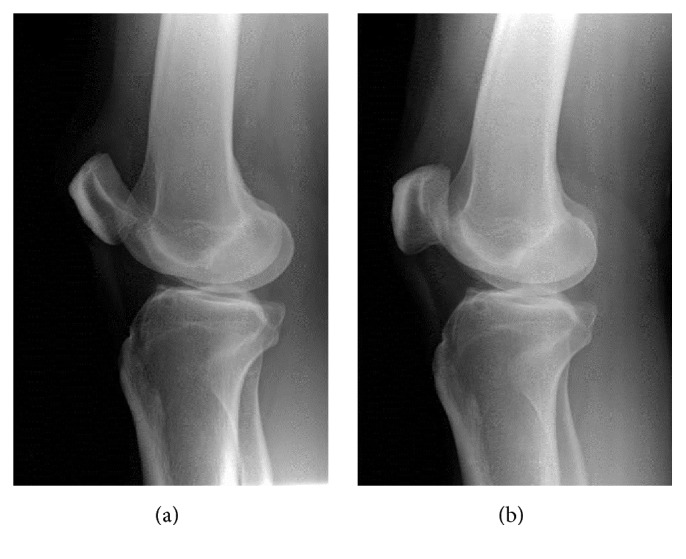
Pre- and postreduction lateral radiographs show superior dislocation of the patella. (a) showed high-riding patella with inferior patellar osteophyte locked to osteophyte at the superior aspect of femoral condyle. (b) showed normal position of patella.
